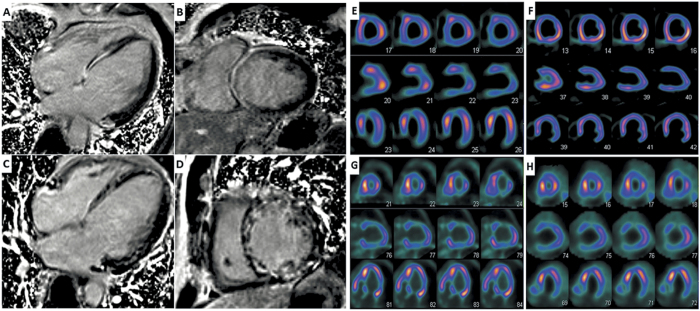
Representative examples of LGE-positive and perfusion/metabolism abnormality images. The typical LGE pattern in DCM is mid-wall enhancement in the interventricular septum (A,B). A diffuse pattern was observed in 5 out of 31 LGE-positive patients (C,D). Severely reduced uptake of ^99m^Tc-MIBI in apex, anterior and inferior wall (E) while ^18^F-FDG PET imaging showed normal FDG uptake (F) in corresponding areas, which was defined as a perfusion/metabolism mismatch pattern. Reduced uptake in anterior, mildly to moderately reduced uptake in lateral and inferior wall in both ^99m^Tc-MIBI SPECT (G) and ^18^F-FDG PET imaging (H), which was defined as a severe perfusion/metabolism match pattern in anterior and mild-to-moderate match pattern in lateral and inferior wall.
